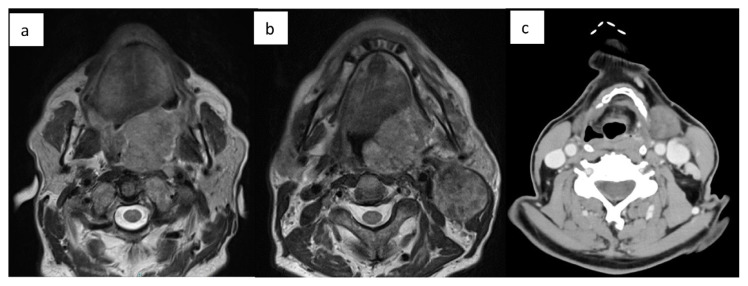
MRI and CT axial images before chemotherapy. a) MRI image with an axial cut on the lingual plane; b) MRI image with an axial cut on the mandibular plane; c) CT image with an axial cut on the hyoid bone plane.
